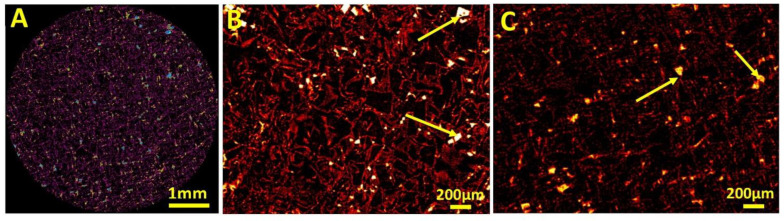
(A–C) The reconstructed cross-section of P(3HO) patch, obtained through the µ-CT method. Yellow arrows indicate porogen grains present in the prepared material.
